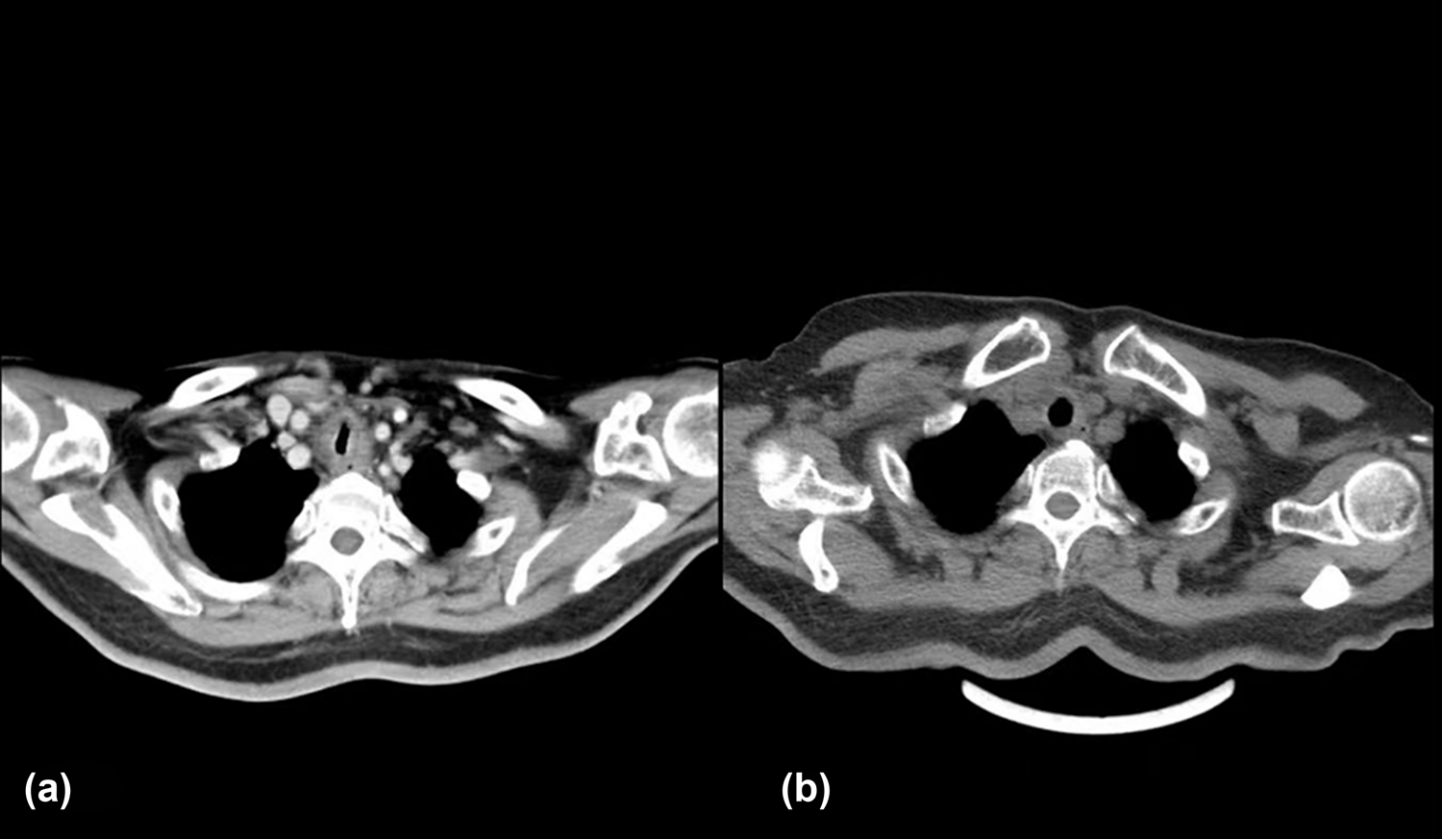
CT scan of tracheostenosis before (a) and after (b) changing the treatment regime for RS with breast involvement and tracheal invasion. (a) Manifestations of severe tracheostenosis. (b) Significant improvement in tracheostenosis.
